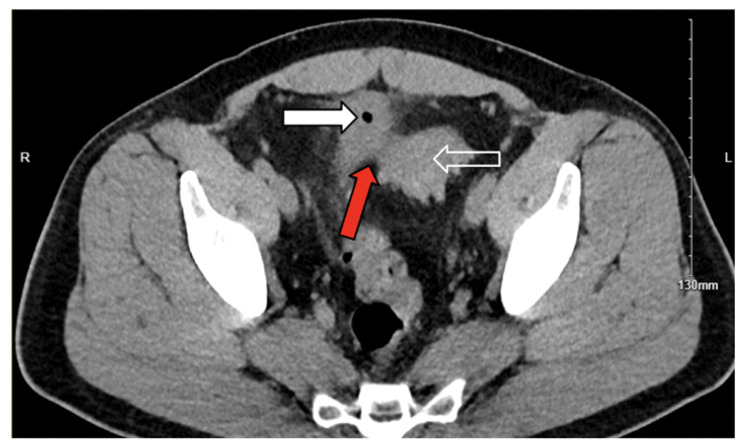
Axial noncontrast computed tomography image demonstrating confluent fat stranding and inflammation (red arrow) between the sigmoid colon (white outlined arrow) and left superior bladder wall. White arrow: air within bladder.
